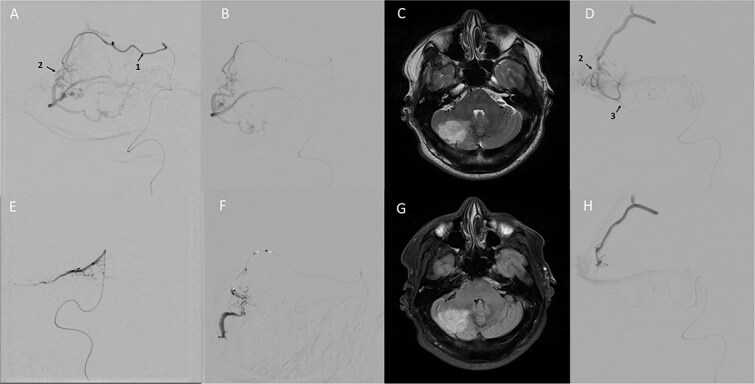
First and second stages of cerebellar AVM embolization. A, B - DSA in lateral view shows contrasting of the subtentorial AVM from the afferent of the right superior cerebellar artery; E,F - first stage of embolization in frontal (E) and lateral (F) views, the images show the spread of the embolic agent - Squid 12,18 (Balt); C,G - postoperative brain MRI (C- axial T2 weighted MRI, G - axial T1 weighted MRI) showing the area of cerebellar ischaemia developed after the first stage of embolization; D - DSA in lateral view showing contrasting AVM from the afferent of the right PICA; H - second stage of total embolization of AVMs from the afferent of the right PICA, the image shows the spread of the embolic agent - Squid 18 (Balt). Black arrows indicate: 1 - afferent from superior cerebellar artery; 2 - nidus of cerebellar arteriovenous malformation; 3 - afferent from the right PICA.
